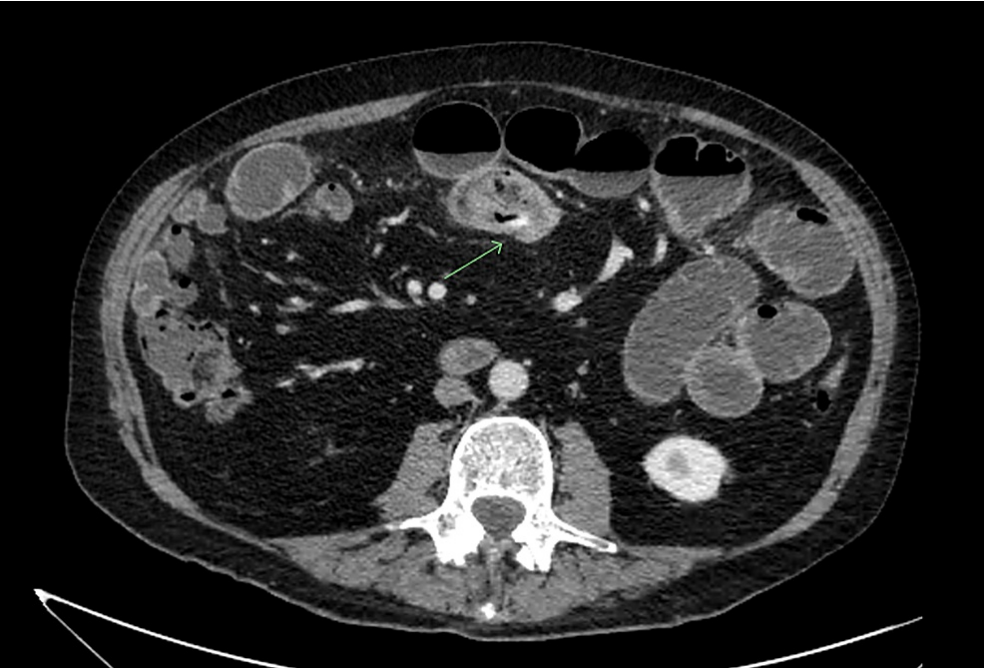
Contrast-enhanced CT scan again demonstrating a transition point but raising concerns of intraluminal abnormality such as intussusception or a foreign body.
